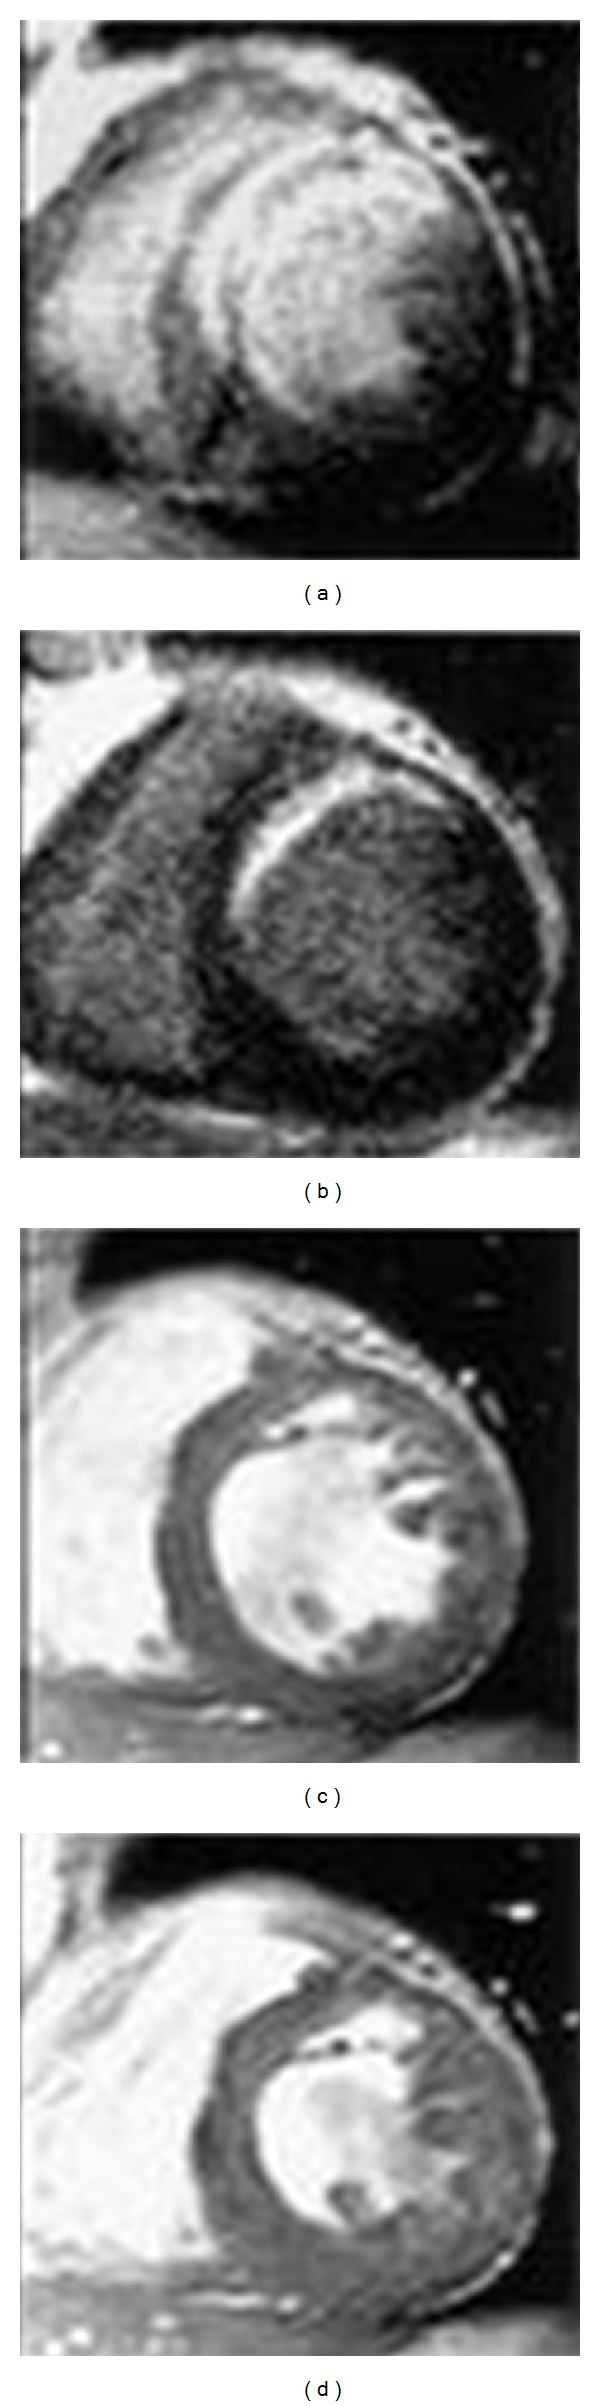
Contrast-enhanced magnetic resonance images in the acute state (a) and chronic state (b), and cine images in the chronic state (c, diastole; d, systole) in Patient who sustained an anteroseptal myocardial infarction. The contractility of the anteroseptal wall was not improved in the chronic state in this patient (with permission from Ichikawa et al.(2005), Am Coll Cardiol, Elsevier Inc., [90]).
